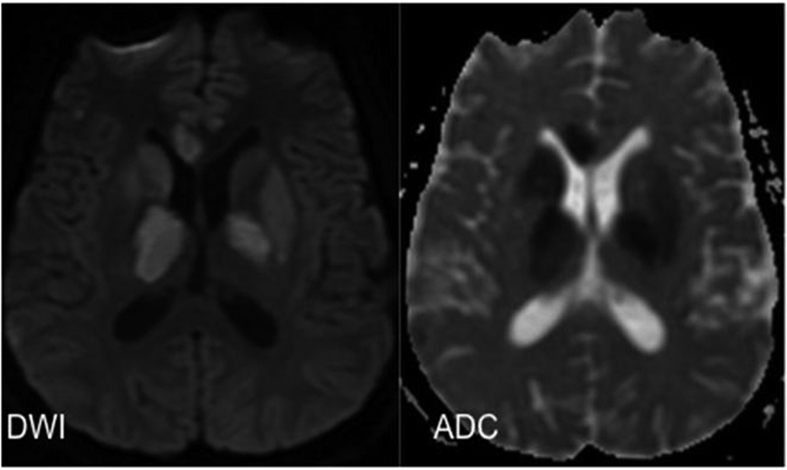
MRI brain ADC/DWI show restricted diffusion in the right caudate nucleus, both thalami, both lentiform nuclei and genu of right corpus callosum, consistent with acute infarction.
